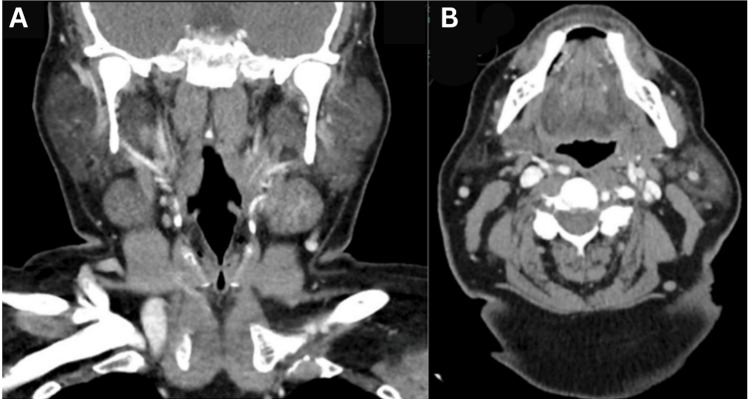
Neck CT with contrast in a patient with a suspected foreign body after consumption of catfish. No foreign body was identified on imaging as demonstrated above. A) Coronal view neck CT with contrast. B) Transverse view neck CT with contrast.
